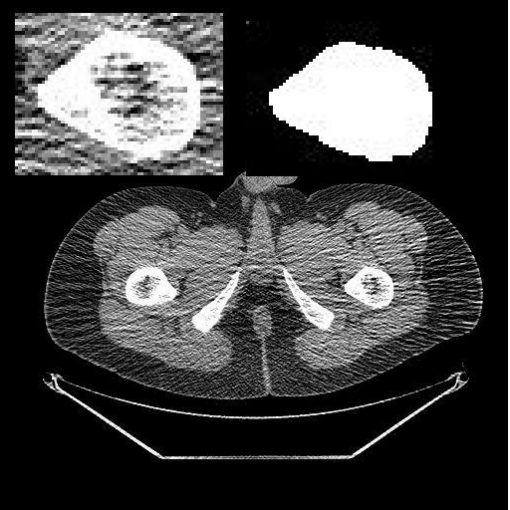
The computer model of rotational osteotomy from CT. The original CT slice is shown. (a) On the left top, we choose the interest region (around femur). (b) The right top shows the result of image processing. (c) The computer binarizes the picture, removes the isolated pixels, fills the medullary canal, and then calculate the gravity center and the errors.
